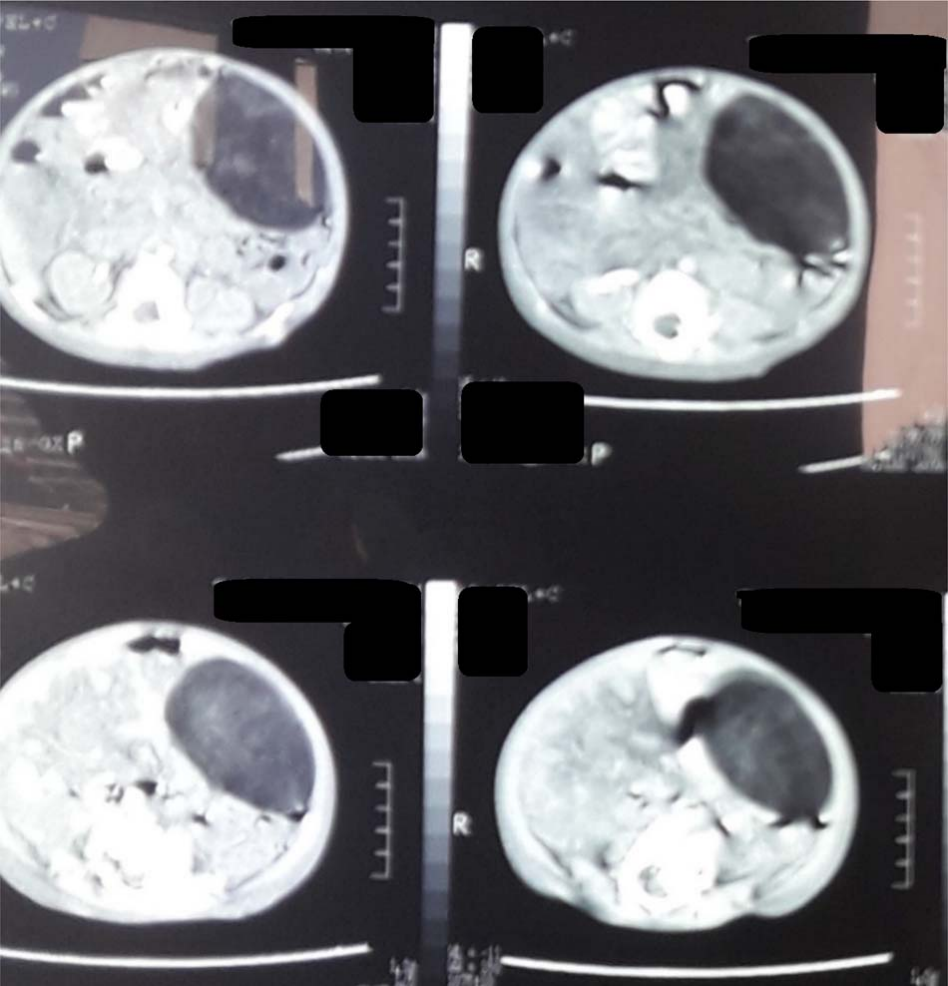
CT scan of the abdomen revealed a fat density mass with defined margins filling the left abdomen and shifting the small bowel to the right.
